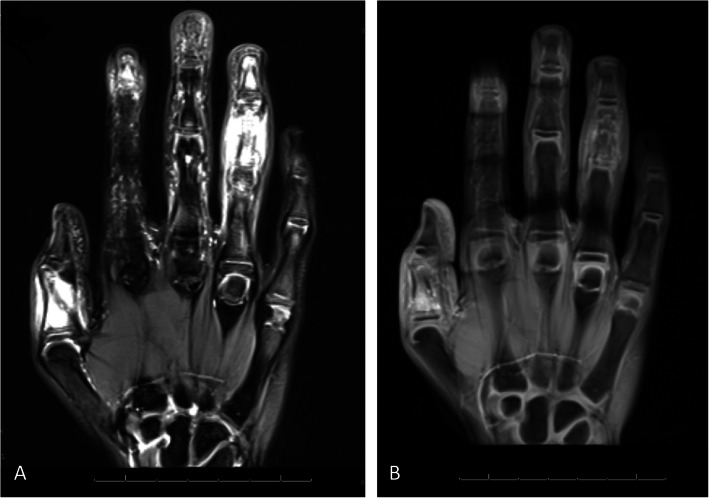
Right hand MRI a. Coronal proton density fat saturation sequences of the right hand shows increased water signal involving multiple phalanges, but most prominently the first proximal phalanx and fourth middle phalanx. Abnormal marrow signal is associated with periosteal lifting and soft tissue edema. b. Coronal T1 post-contrast with fat saturation shows contrast enhancement of the marrow space and soft tissue. Note the absence of joint effusions and synovial enhancement
